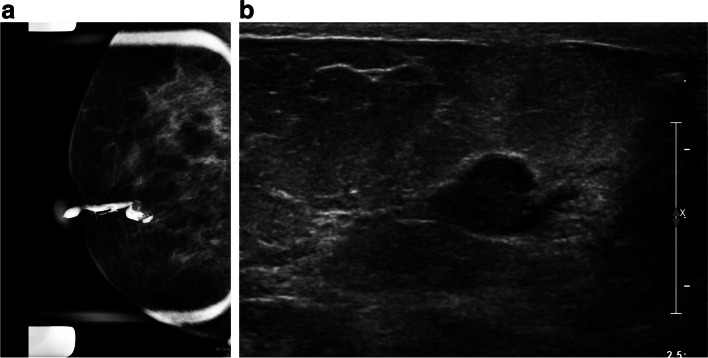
A 43-year-old woman with bloody nipple discharge and several likely benign masses in her US underwent ductography via cannulation of the duct with discharge. Ductography helped to find the pathology. Ultrasound-guided biopsy of the mass showed benign papilloma
